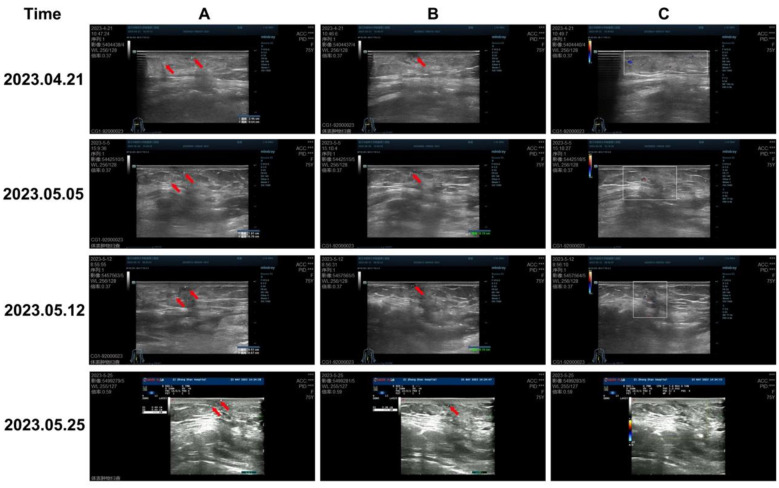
Size changes of incisional infection site on ultrasonography. (A) means the length and width of the incisional infection site. (B) means the width of tube to skin. (C) means the blood flow in the incisional infection site.
